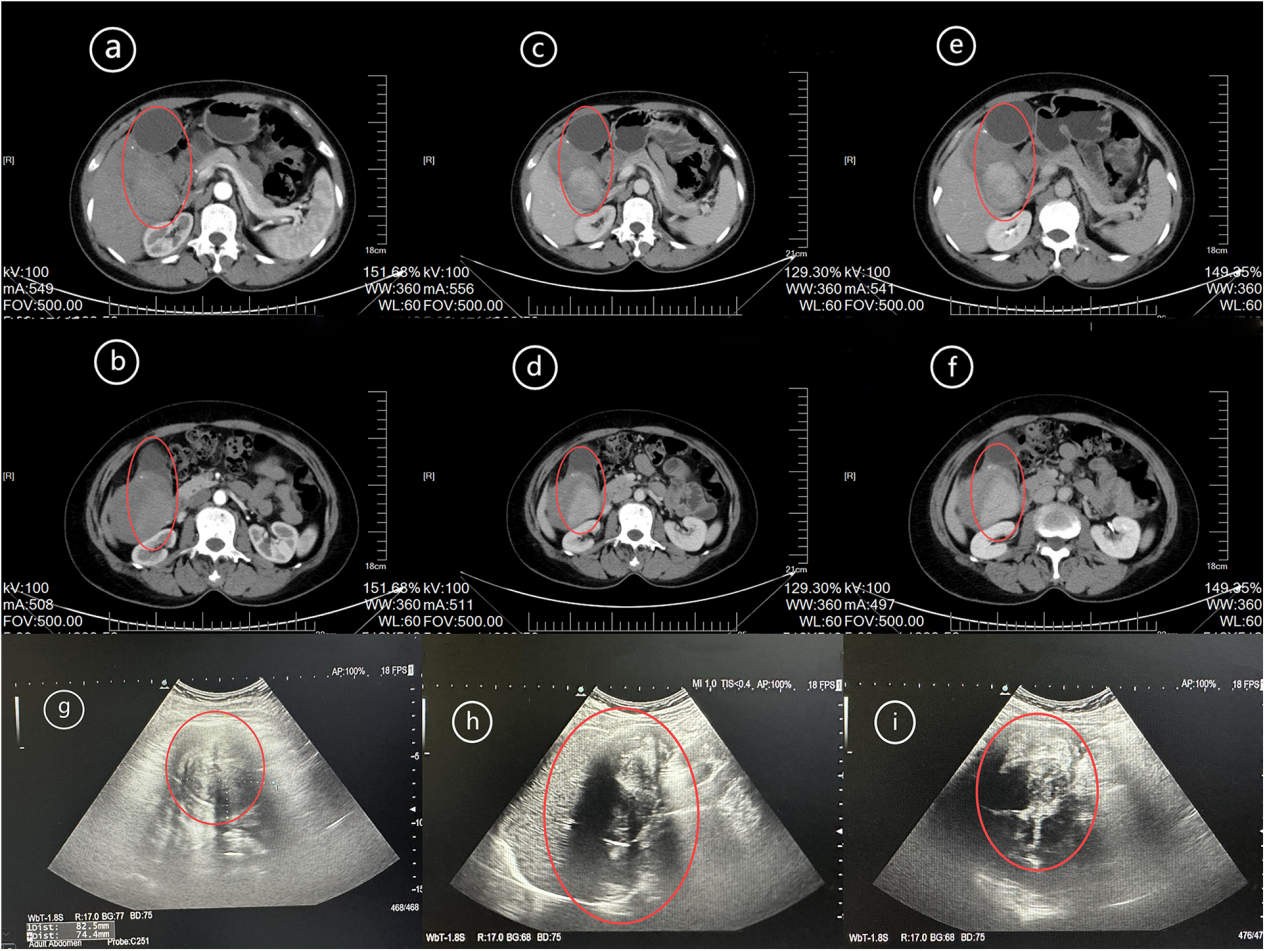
Radiological findings: the patient's dedifferentiated liposarcoma. Hepatobiliary, splenic, and pancreatic multi-row CT scan + three-phase enhancement: arterial phase (a,b), portal phase (c,d), and equilibrium phase (e,f) suggest an irregular large mass-like soft tissue shadow, with non-uniform density and a small amount of nodular calcification, and the enhancement scan shows obvious non-uniform enhancement (red circle); abdominal ultrasound (g–i) suggests a confined solid, strongly and weakly echogenic cluster, with non-uniform internal echogenicity, and irregular morphology (red circle).
